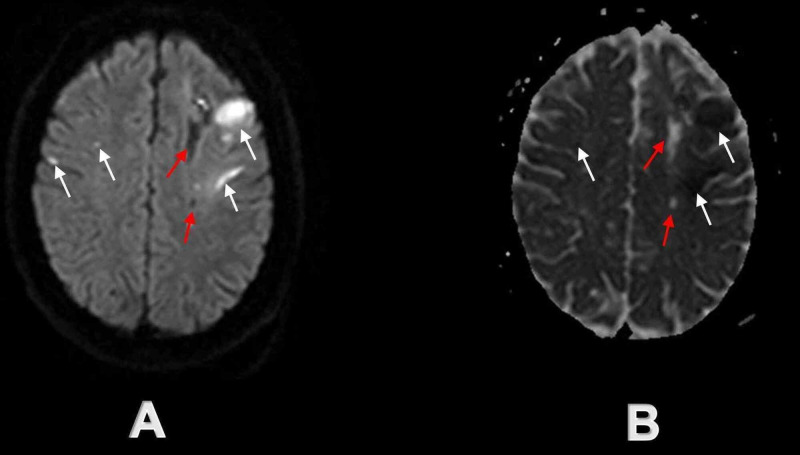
Initial brain MRI at the time of admission demonstrating acute and chronic infarcts in bilateral high frontoparietal lobes White arrows demonstrate multiple acute ischemic infarcts on axial DW sequence (A) and axial ADC sequence (B) in bilateral high frontoparietal lobes, while red arrows demonstrate simultaneous chronic ischemic infarcts on axial DW sequence (A) and axial ADC sequence (B) in the centrum semi-ovale mostly on the left side. DW - diffusion-weighted; ADC - apparent diffusion coefficient
